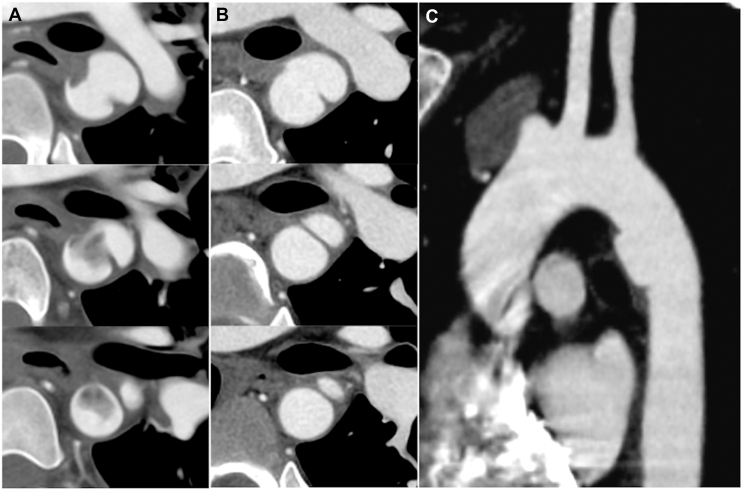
Preoperative computed tomography images. (A) An aortic injury is observed at the aortic isthmus with some blood clots within the aortic wall. (B) These disappeared at the 1-month follow-up examination. (C) The aortic injury is located on the lesser-curvature side.
